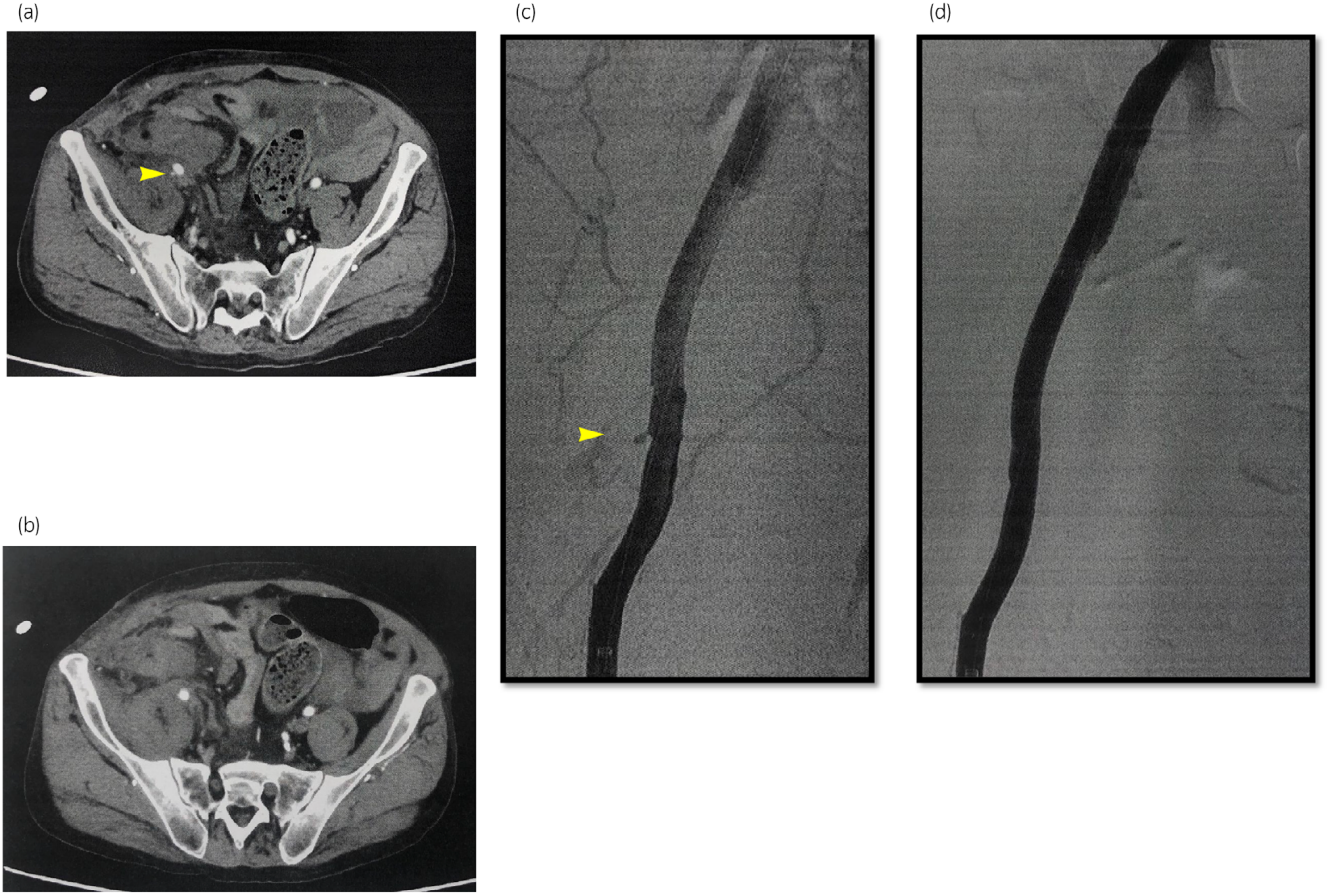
Contrast test to identify the bleeding site. (a) CT showing a pseudoaneurysm at the anastomosis of the EIA (b) The contrast material is also exposed to the abdominal cavity (c) Angiography showing the pseudoaneurysm (d) Covered stent placement (thickness, 8 mm; length, 10 cm).
